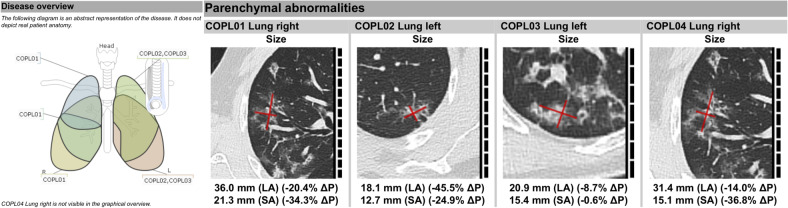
Overview of locations of major findings on chest CT. Longitudinal overview of documented findings on chest CT examination (here: evaluation after second chest CT in COVID-19 disease course). Decrease in the size (LA long axis, SA short axis) of the imaging findings. These findings are included in an automatically generated case report file for a COVID-19-positive patient and study data repository.
